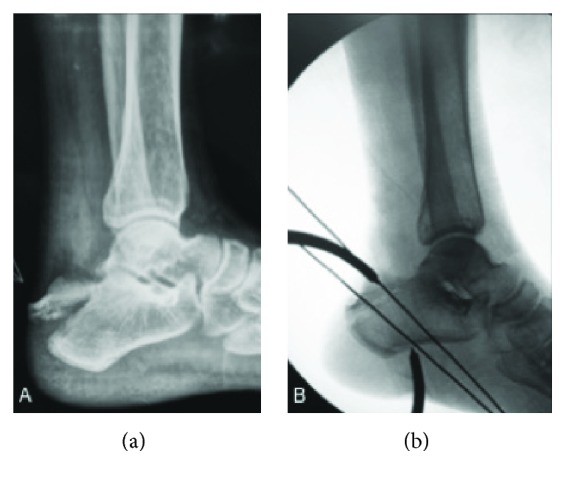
(a) Left lateral hindfoot radiograph shows an extra-articular calcaneal avulsion fracture occurring in conjunction with a fracture of the medial process (Beavis II, Squires I). Note the proximal fragment pulled cephalad by the Achilles tendon. (b) Interfragmentary compression is maintained with a periarticular reduction clamp, and provisional fixation is performed with two 2.0 mm K-wires inserted from the superior aspect of posterior tuberosity of the calcaneus.
